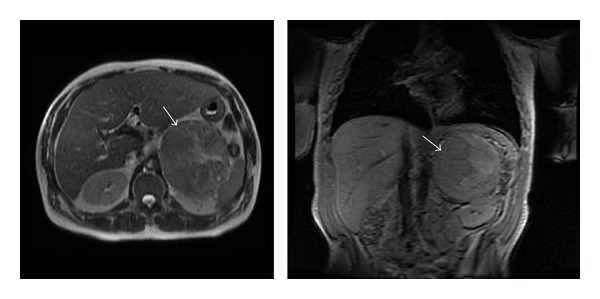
Magnetic resonance image of the abdomen demonstrating the left adrenal mass in transverse and coronal planes.
